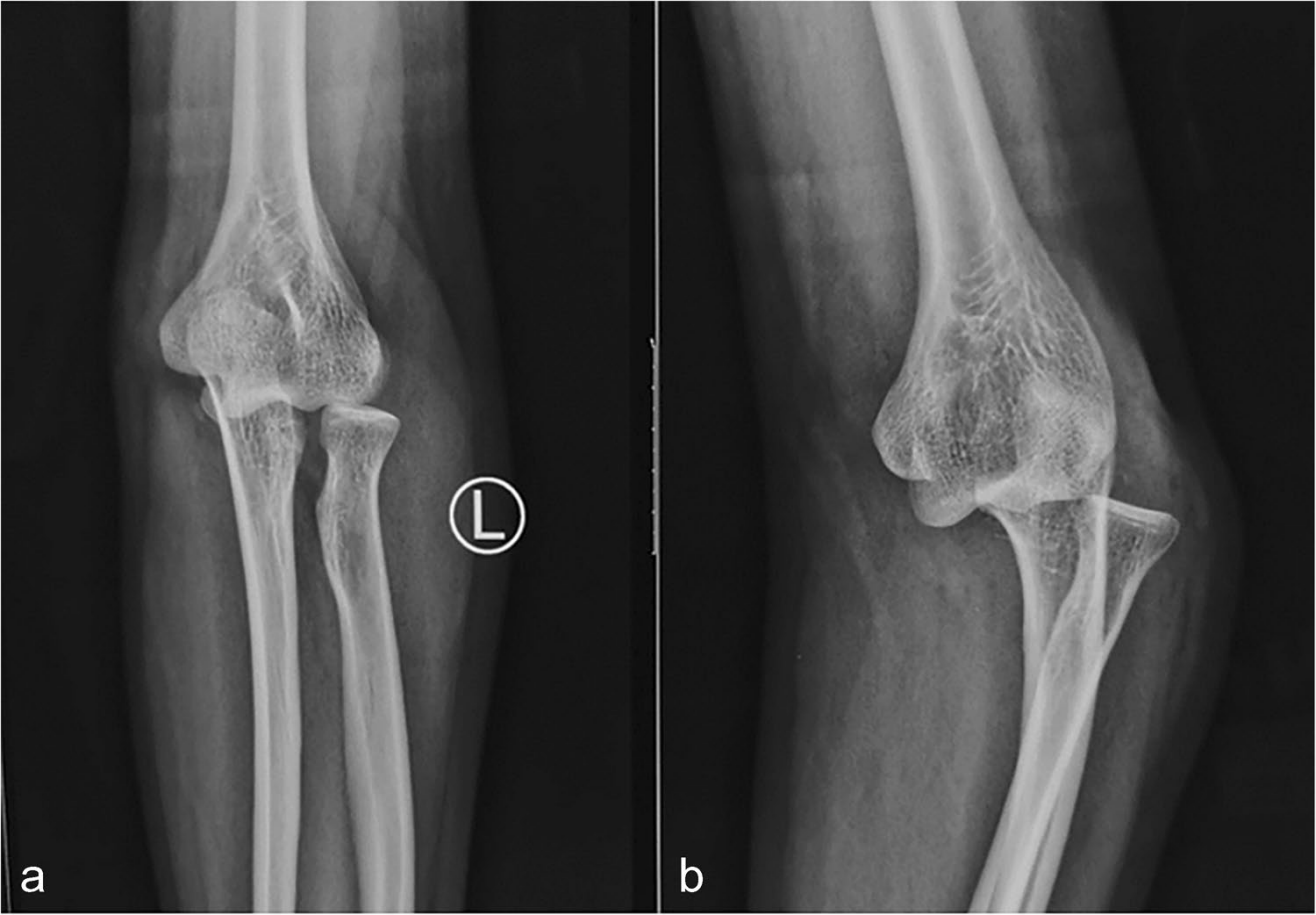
Anteroposterior and lateral X-ray radiographs show an isolated divergent dislocation of the elbow (a–b)
